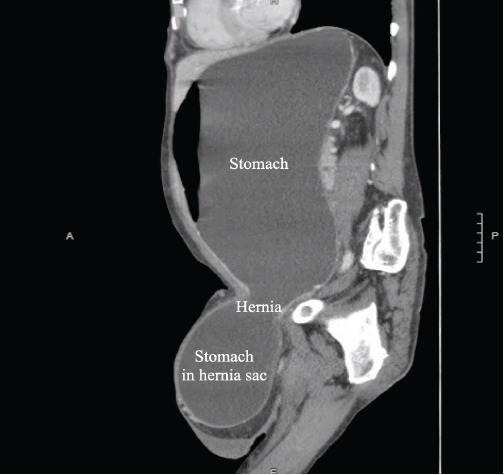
Computed tomography with sagittal images demonstrating a large and distended stomach partially in an inguinal hernia.
